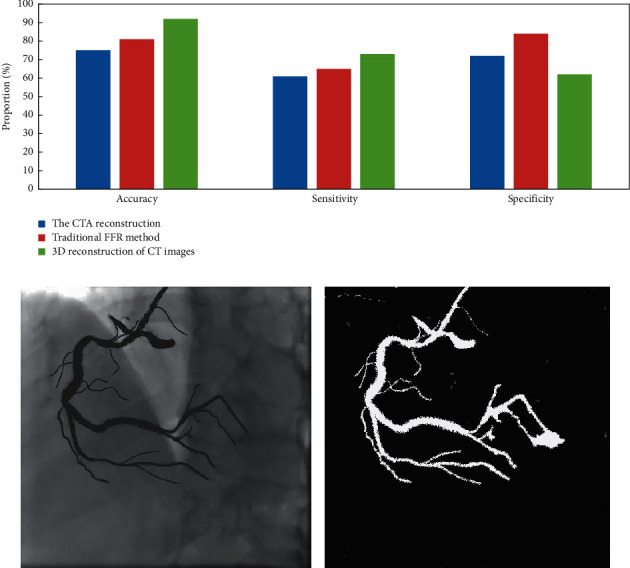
3D reconstruction results of CT images. (a) Comparison of three reconstruction methods; (b) CT image of coronary artery; (c) coronary artery 3D model reconstruction.
